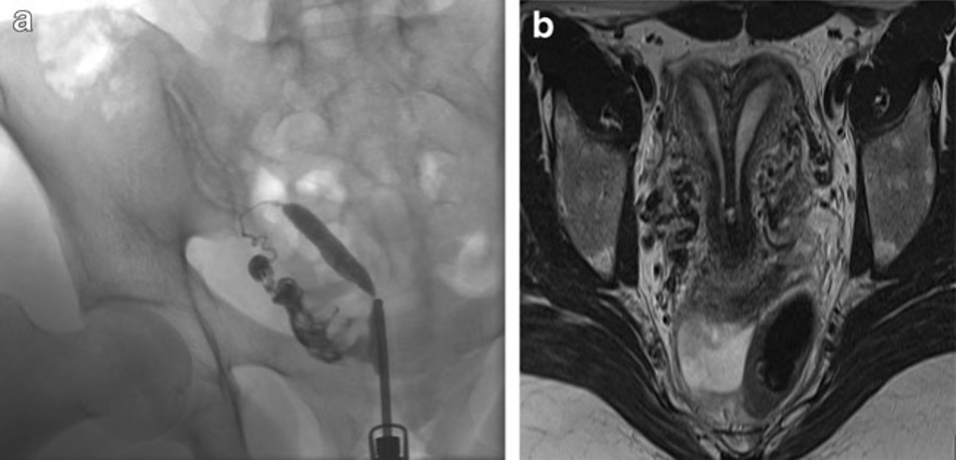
A 31-year-old patient: Suspected uterus unicornis unicollis at HSG (a) was identified as bicornis bicollis with MRI (b). MRI: T2-weighted, transversal oblique MRI of the pelvis demonstrates two uterine cavities separated by a muscular layer. Uterus didelphys was rated unlikely as no vaginal septum was visible with MRI. Laparascopy is planned
